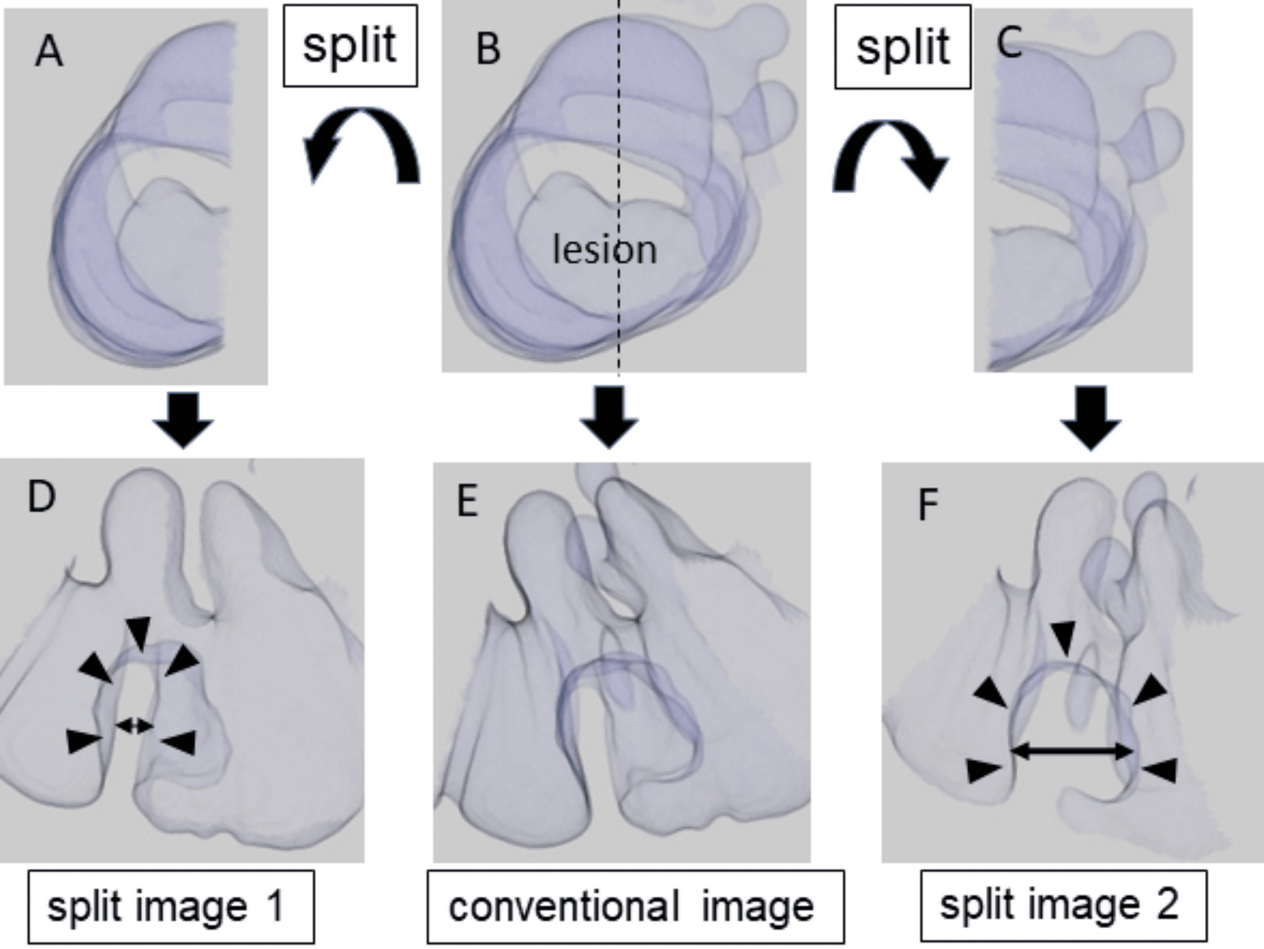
Definition and measurement of the width of colorectal-fold lateral contour using lateral split-view CT enema images of a CRC located on a colorectal fold. First, the split images (A, C) at the central part of the lesion in the cross-sectional view (B) of the CT enema image in the CRCs were extracted. Next, the value of the width (double arrow) of colorectal-fold lateral contour (arrowhead) in the lateral view (D, F) of each split CT enema image was calculated, and the maximum value of the width of colorectal-fold lateral contour was defined as the value of the width of colorectal-fold lateral contour of the CRC located on a colorectal fold. In this case, the value of the width of colorectal-fold lateral contour calculated using split image 2 (F) was defined as the value of the width of colorectal-fold lateral contour of the CRC located on a fold. (E) The lateral view of the conventional CT enema image. This figure was created by the authors for this study. CRC: colorectal cancer; CT enema: computed tomographic air-contrast enema
